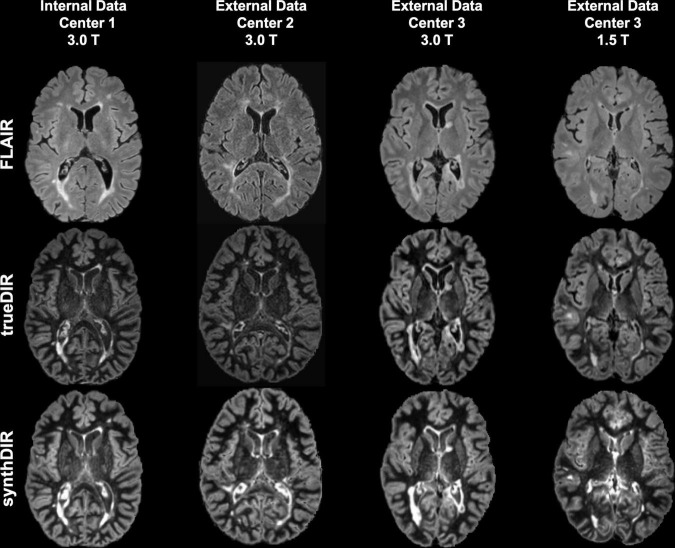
Exemplary images of FLAIR, trueDIR, and synthDIR for all centers and scanners.
